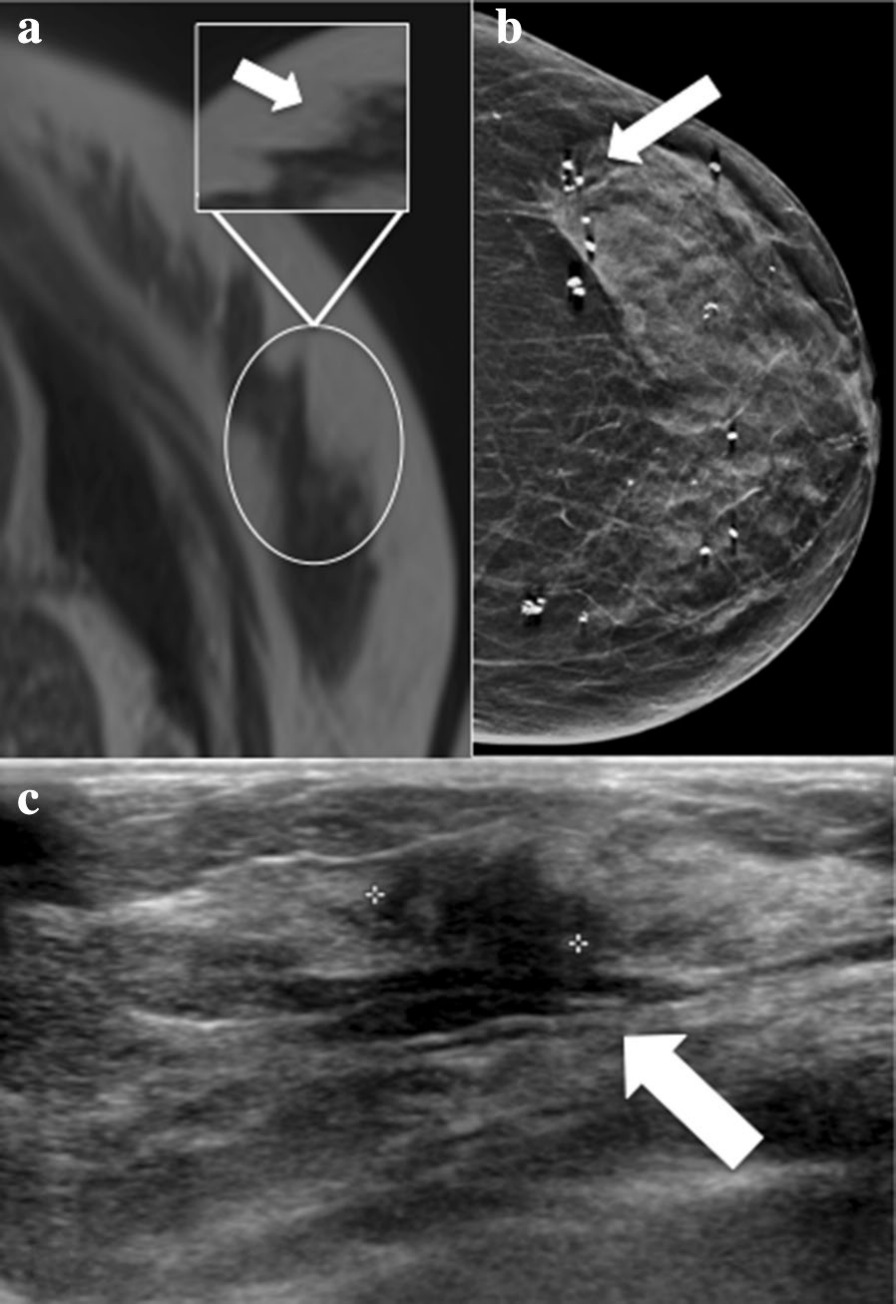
Examples of breast incidental findings by BI-RADS category. a A 75-year-woman (Patient 1 in Table 3) underwent MR cholangiopancreatography. More than 50 % of the breast tissue was visible at abdominal MRI. A suspicious architectural distortion (*white circle*) was found in the outer quadrants of the left breast (BI-RADS RM 4). In the *box* is highlighted the breast findings. b Left full-filed digital mammography shows an area of architectural distortion (*white arrow*) in the outer quadrants. c Corresponding US *image* shows a mass with indistinct borders in the left breast at 2 o’clock position (15 mm diameter). The US-guided core needle biopsy confirmed a B5b lesion (invasive ductal carcinoma)
